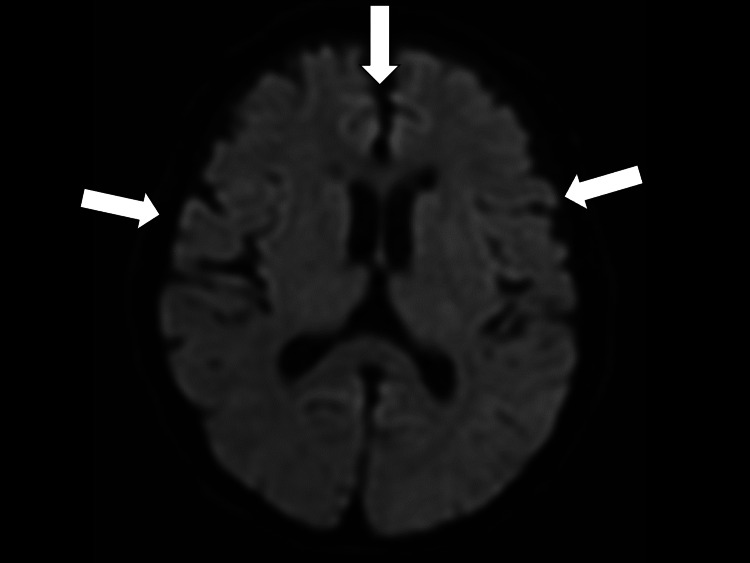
Magnetic resonance imaging (diffusion) showing a high pericerebellar signal (white arrows)
